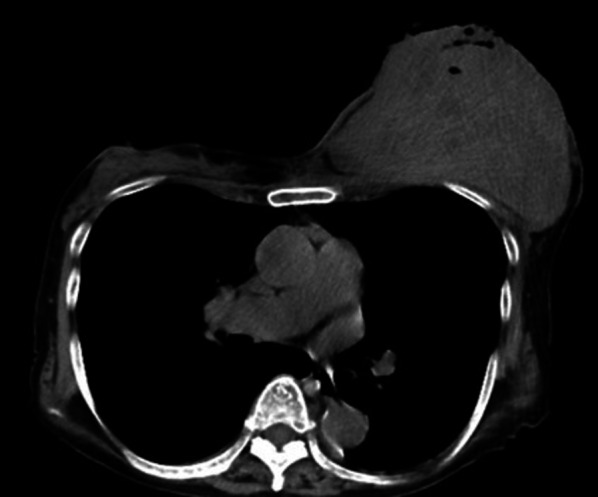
Breast computed tomography image. A 12 × 10-cm-sized mass was found in the left breast. No obvious pectoralis major muscle invasion was observed. Additionally, there was no significant lymph node swelling or findings suspicious of distant metastasis
